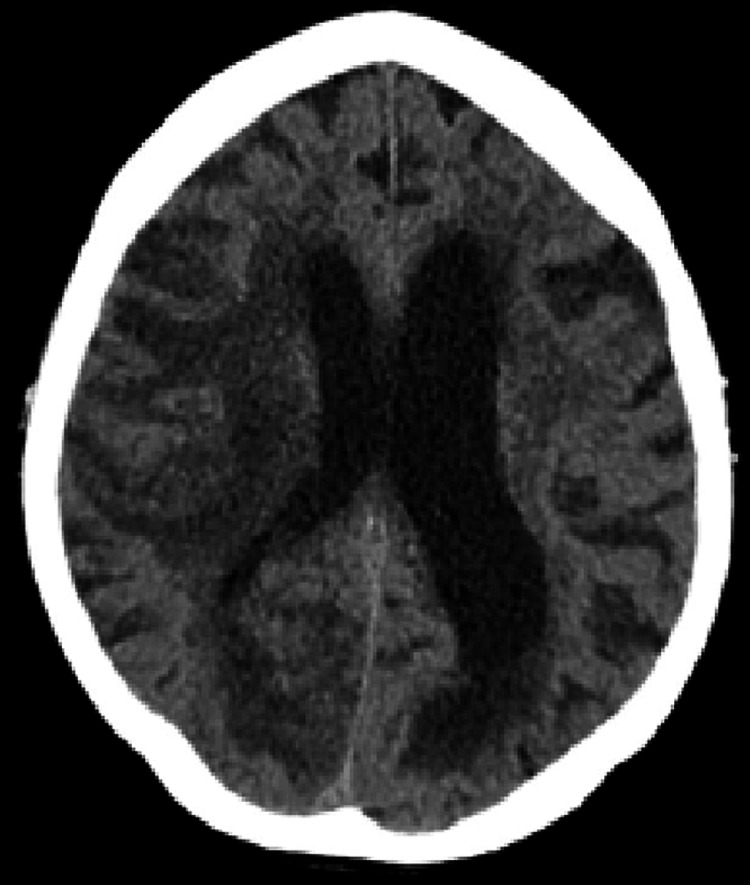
Day 4. Axial CT brain demonstrating widespread vasogenic oedema in the right frontal, temporal, and parietal lobes. Moderate mass effect with effacement of the posterior right lateral ventricle with associated midline shift. Features of early hydrocephalus with distention of the left temporal horn are also demonstrated.
